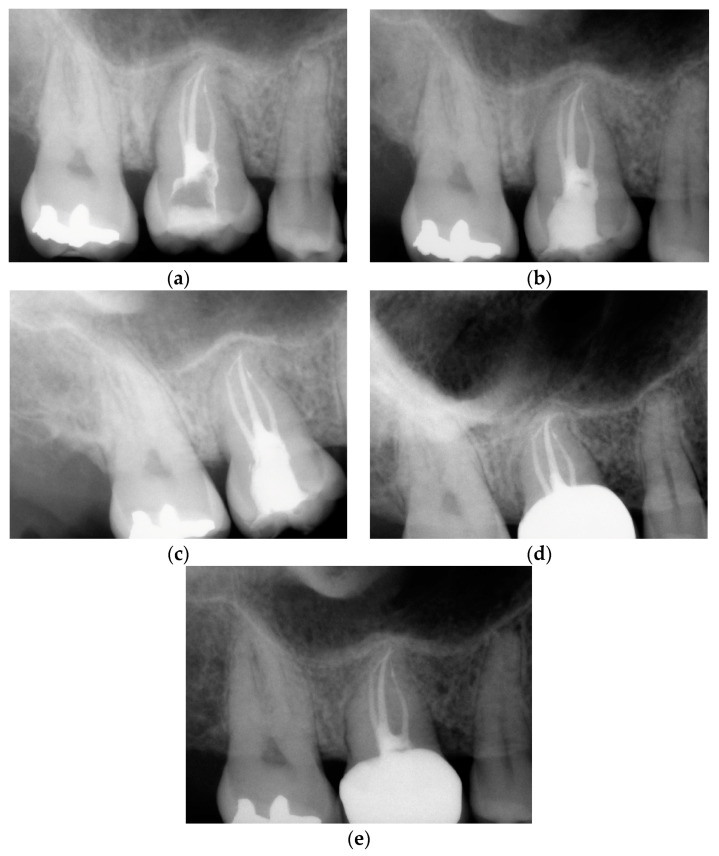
Periapical control radiographs. (a) After 3 months, endodontic therapy was performed; (b) after 6 months; (c) after 12 months; (d) after 18 months, prosthetic coverage with a dental crown in zirconia was applied; (e) after 36 months.
